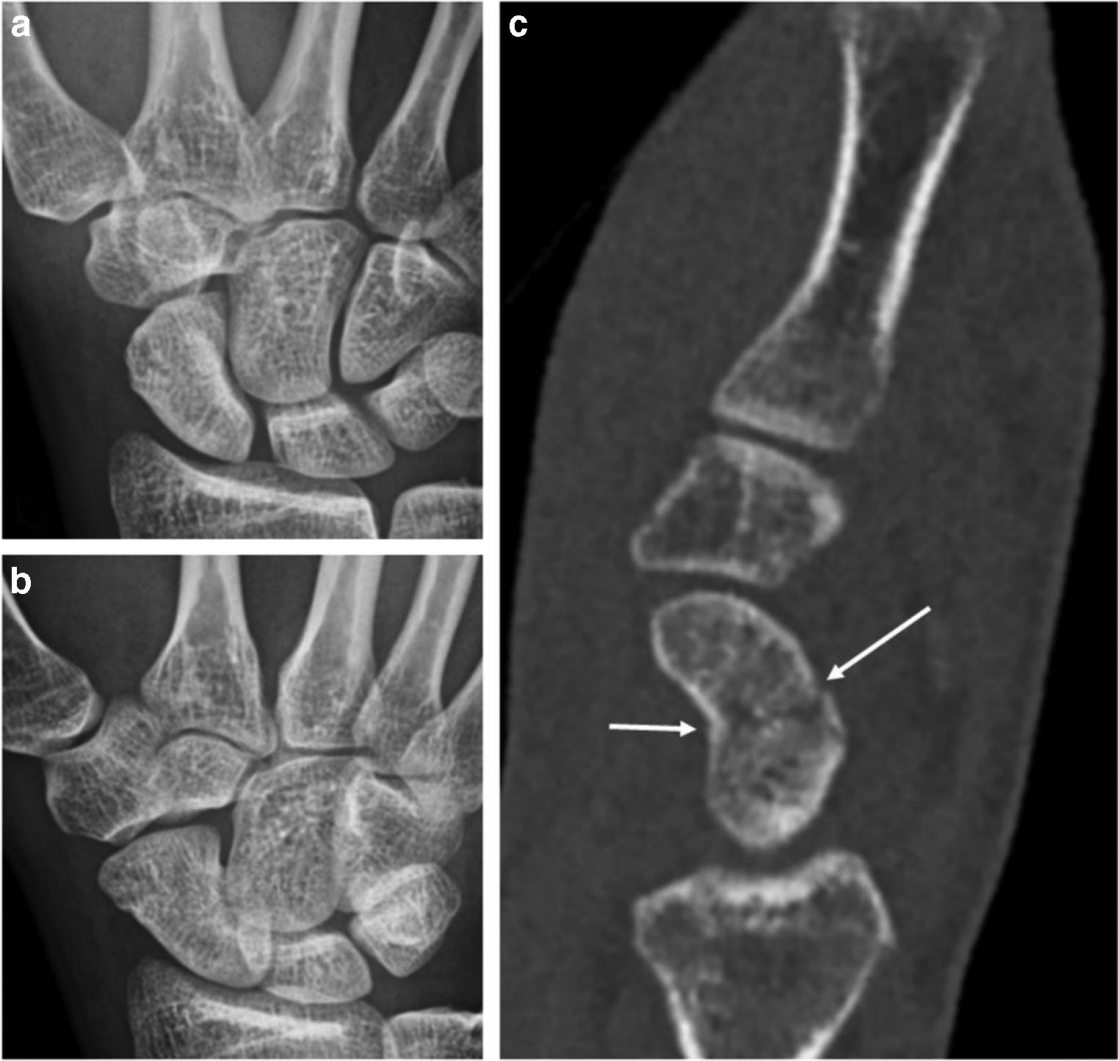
A 29-year-old male fell on an outstretched hand during soccer practice. He experienced instant sharp wrist pain. He presents to the ER with swelling, limited mobility, and local tenderness in the anatomical snuffbox. DR scaphoid projections show no signs of fracture (a, b). Subsequent ULD-CT demonstrates a nondisplaced scaphoid waist fracture (c). The treatment recommendation was upgraded from functional to cast immobilization
